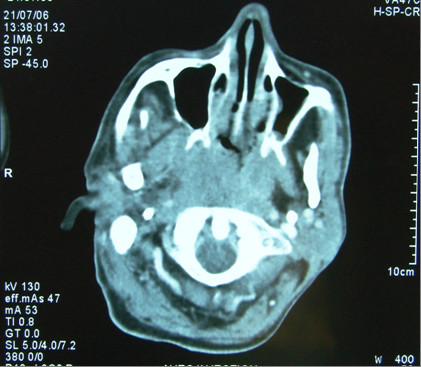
Computed tomography of the head and neck shows the involvement of the nasopharynx by the oropharyngeal process.
